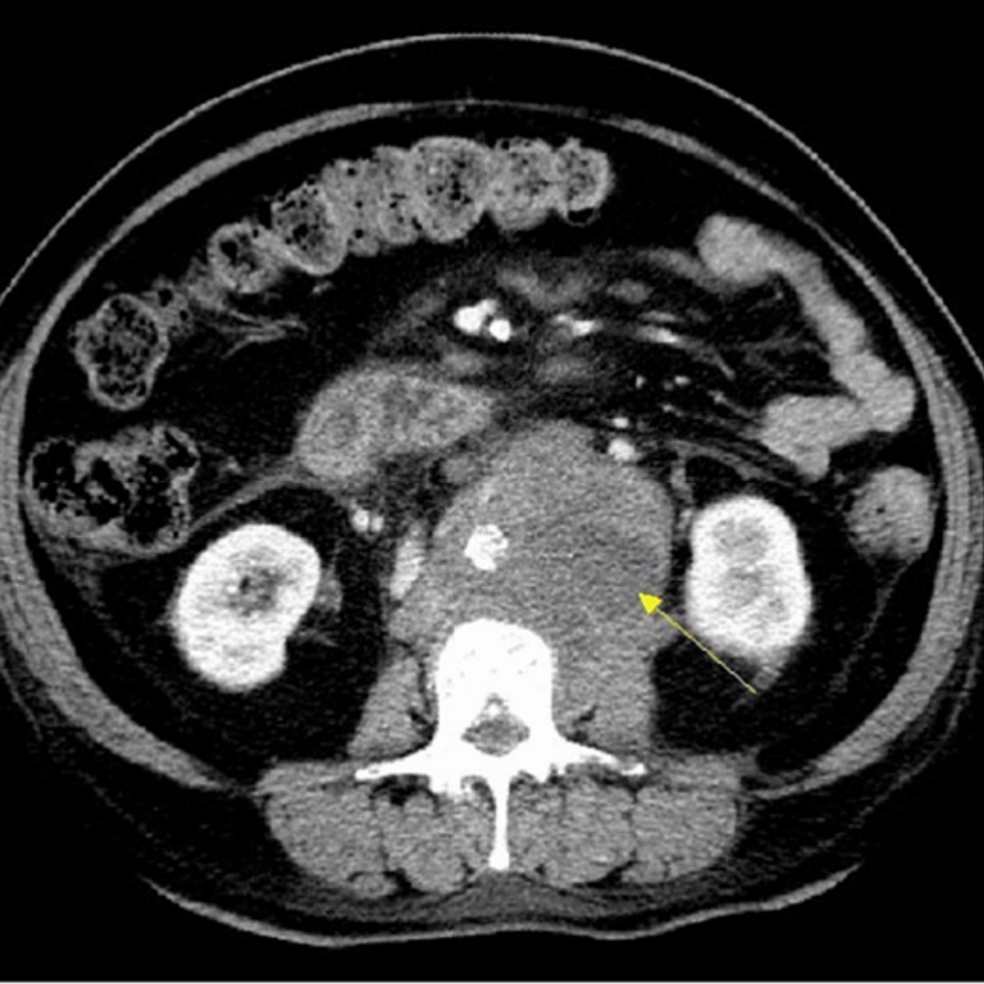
Abdominal CT showing multiple masses anterior to the aorta
